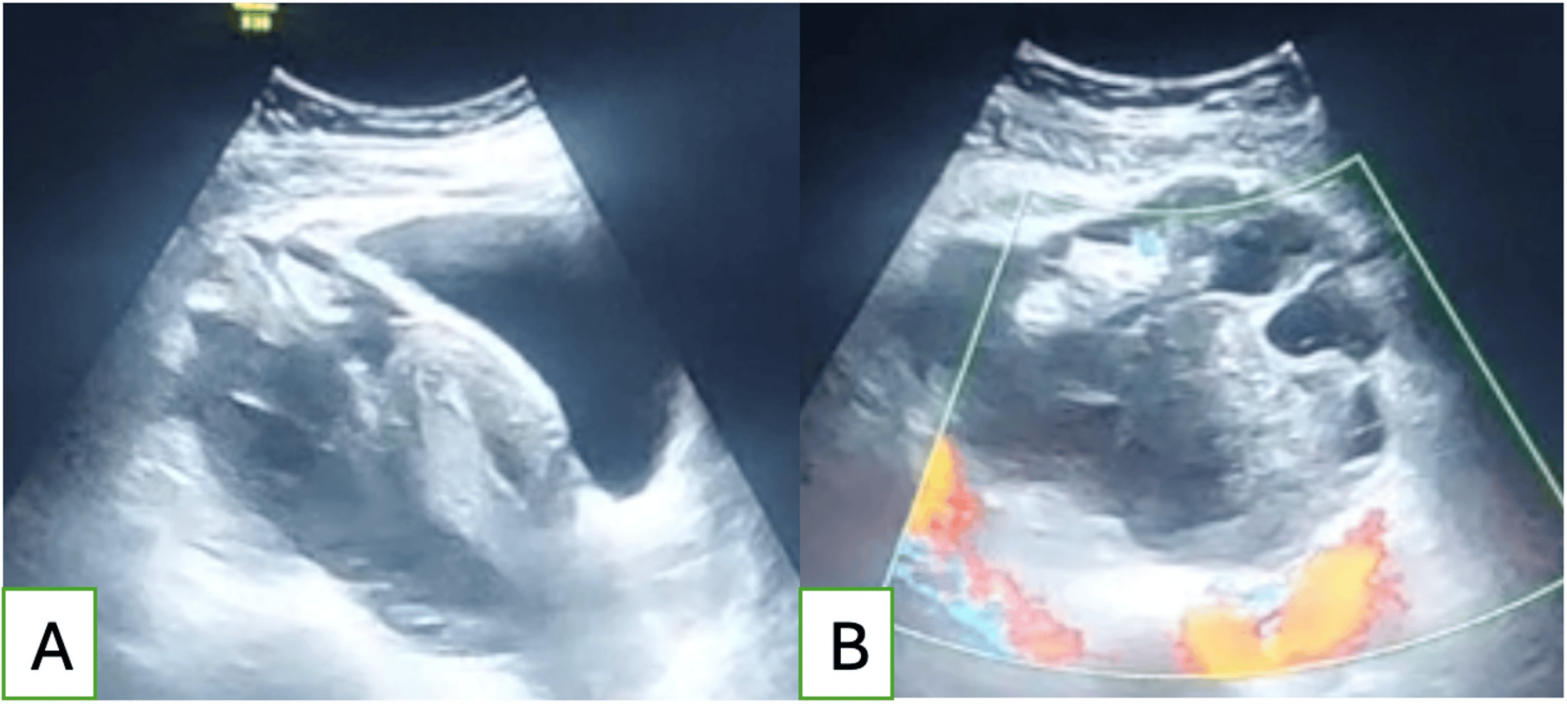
Ultrasound images of a left adnexal mass suggestive of a ruptured hemorrhagic cyst (A) Sagittal view showing the uterus with a hemorrhagic cyst. (B) Axial view of the cyst with color Doppler.
